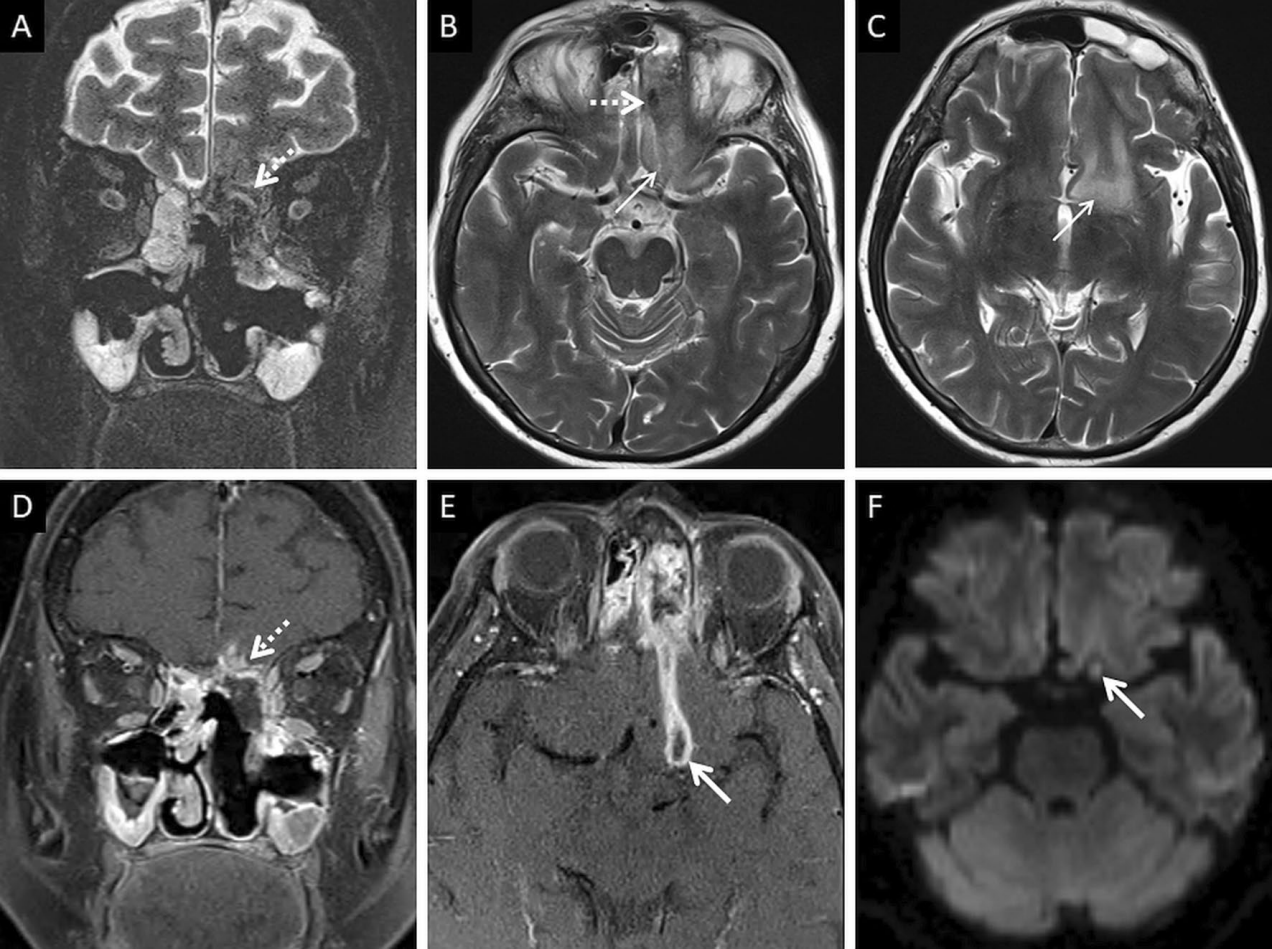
Cerebritis and parenchymal abscess formation in ROCM. Pre-operative imaging workup was performed at another institution. Post-operative MRI, performed at our institution, is presented. Coronal T2-FS image (a) demonstrates ill defined, hypointense soft tissue in the left ethmoid air cells, invading into the left anterior cranial / left olfactory fossa (dotted arrow). Axial T2W images of the brain (b, c) demonstrates the hypointense soft tissue (dotted arrow in B) along with vasogenic oedema in the left basifrotnal white matter (thin arrows in b, c). CE FS coronal T1W image (d) shows abnormal parenchymal enhancement (dotted arrow) of the left gyrus rectus suggesting cerebritis, due to fungal invasion. CE FS axial T1W image (e) shows a peripherally enhancing lesion (thick arrow) along the posterior portion of the left gyrus rectus. Axial diffusion weighted image (f) shows restricted diffusion within the peripherally enhancing lesion (thick arrow), suggesting abscess formation
